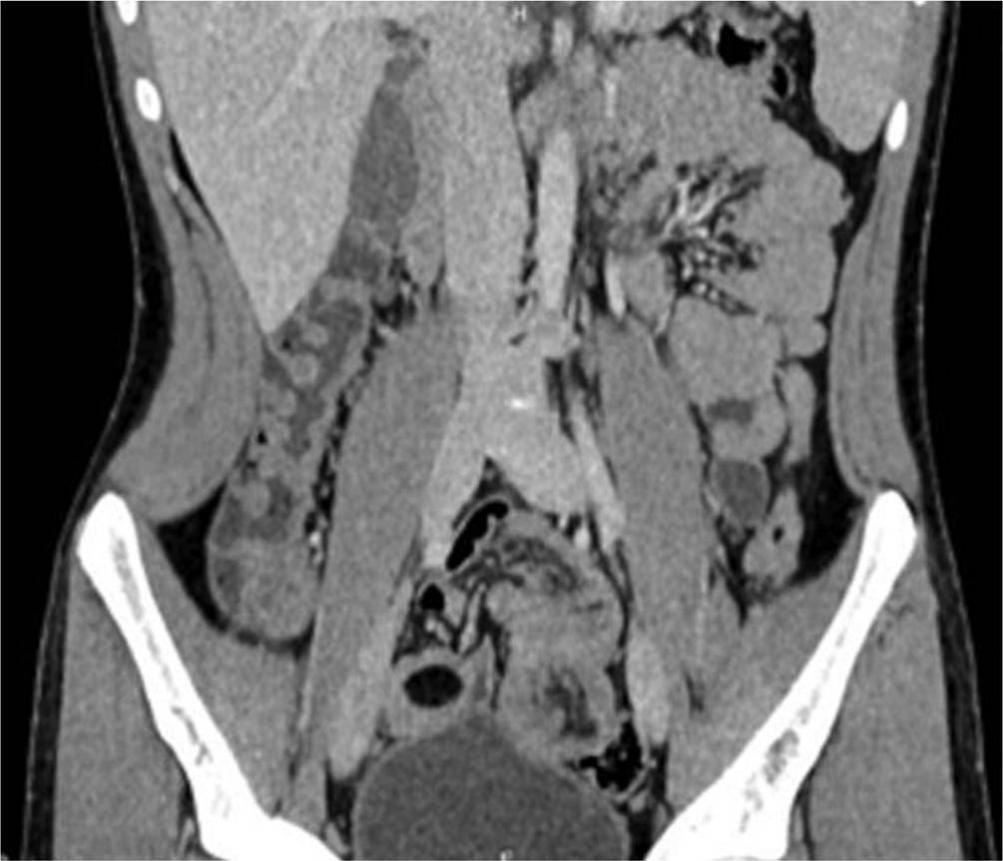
Coronal CT scan showing “sausage-shaped” mass.
